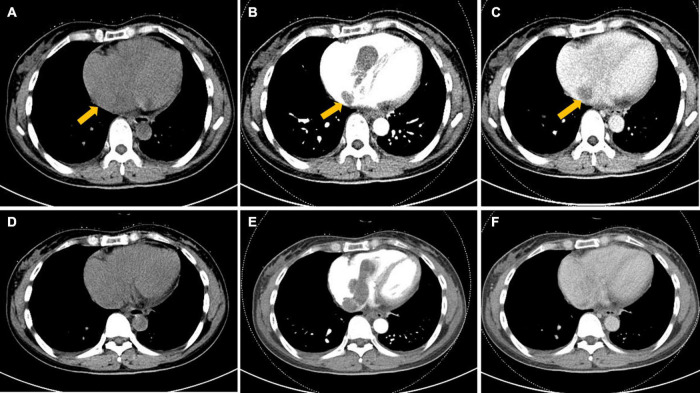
Axial computed tomography (CT) image of the heart. (A–F) Hypoattenuating filling defect located within the right atrium across the tricuspid valve into the right ventricle. Intracardiac lesions demonstrated mainly moderate progressive enhancement, with localized non-enhancing necrotic areas (arrow) on contrast-enhanced CT. (A,D) Unenhanced phase image. (B,E) Arterial phase of contrast enhancement image. (C,F) Portal phase of contrast enhancement image.
